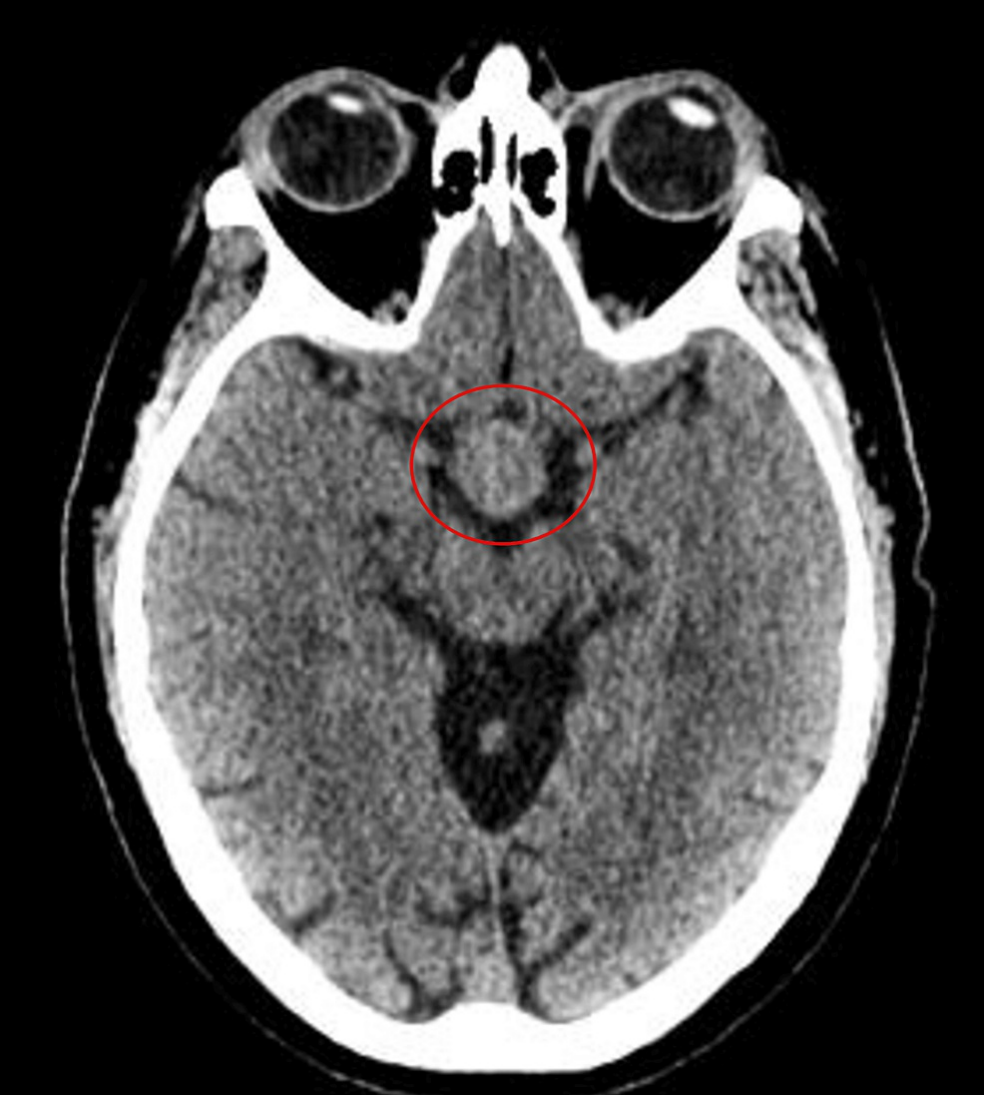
CT brain scan showing no evidence of haemorrhage or ischaemia and a large sellar mass measuring 2.9x2.7x4.1 cm
